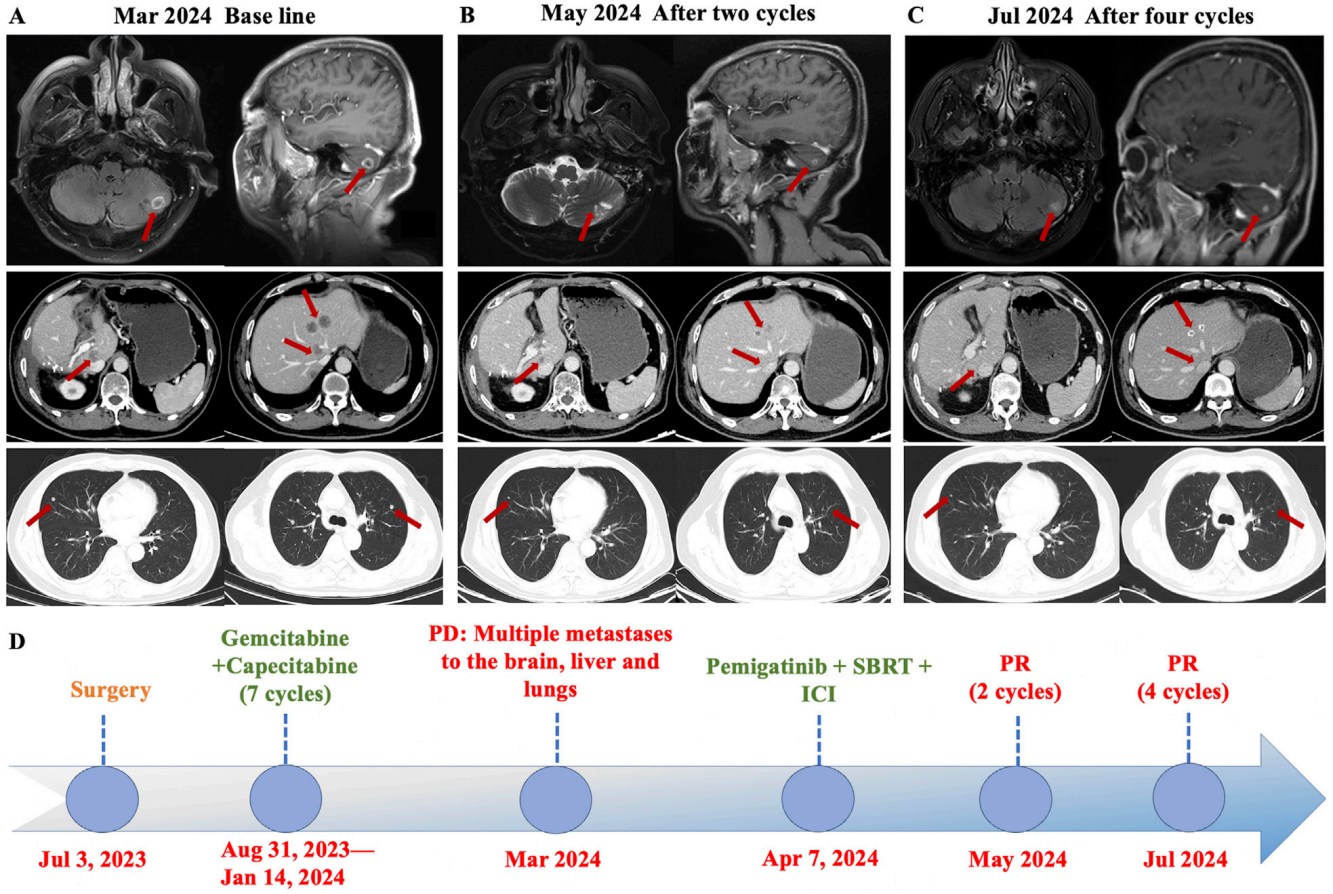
Axial CT images demonstrated changes in the patient’s brain, liver, and lung metastases at baseline and after treatment with pemigatinib combined with immune checkpoint inhibitor (ICI) and stereotactic body radiation therapy (SBRT). (A) The patient developed multiple brain, liver, and lungs metastases during postoperative adjuvant therapy. (B) The metastases in each organ were significantly reduced after two cycles of pemigatinib combined with ICI and SBRT, and the efficacy was evaluated as partial response (PR). (C) After four cycles of the combined therapy, the tumor shrank further and the efficacy was evaluated as sustained PR. (D) Timeline of the disease course and treatment. PD: Progressive Disease, PR: partially reduced, SBRT: stereotactic body radiation therapy, ICI: immune checkpoint inhibitor.
